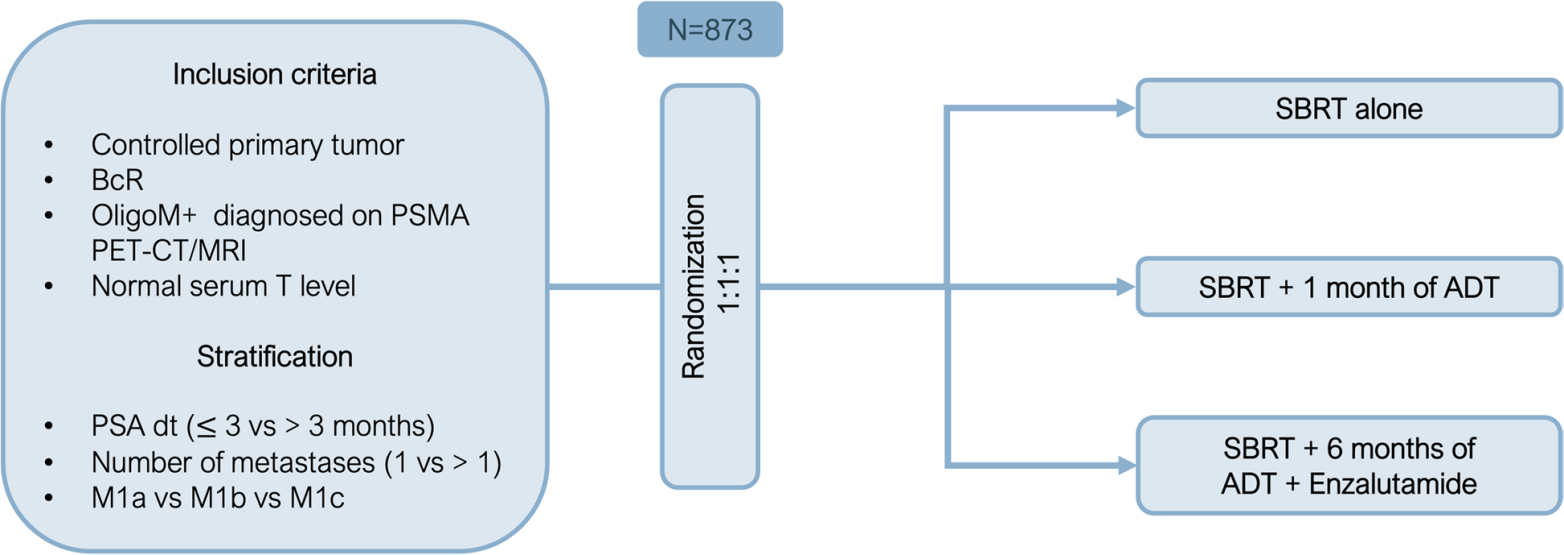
Trial design BcR: Biochemical recurrence; OligoM + : oligometastatic disease; PSMA PET-CT/MRI: prostate-specific membrane antigen (PSMA) positron emission tomography/computed tomography (PET/CT) or positron emission tomography/magnetic resonance imaging (PET/MRI); T: testosterone; PSA: prostate specific antigen; M1a: non-regional lymph node metastases, M1b: bone metastases, M1c: visceral metastases; SBRT: stereotactic body radiotherapy; ADT: androgen deprivation therapy
